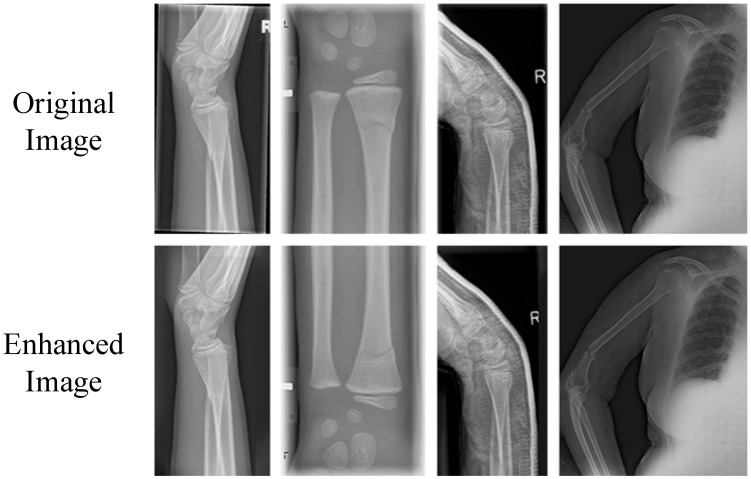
Sample Display of Augmented X-ray Fracture Dataset.
